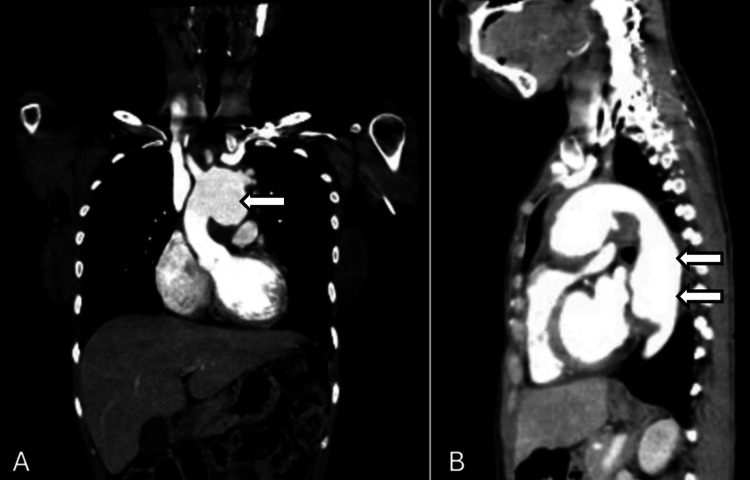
Initial CT angiography showing dilation of the aortic arch, descending thoracic aortic aneurysm and involvement of bilateral carotid and subclavian arteries A: coronal plane. B: Sagittal plane CT: computed tomography
